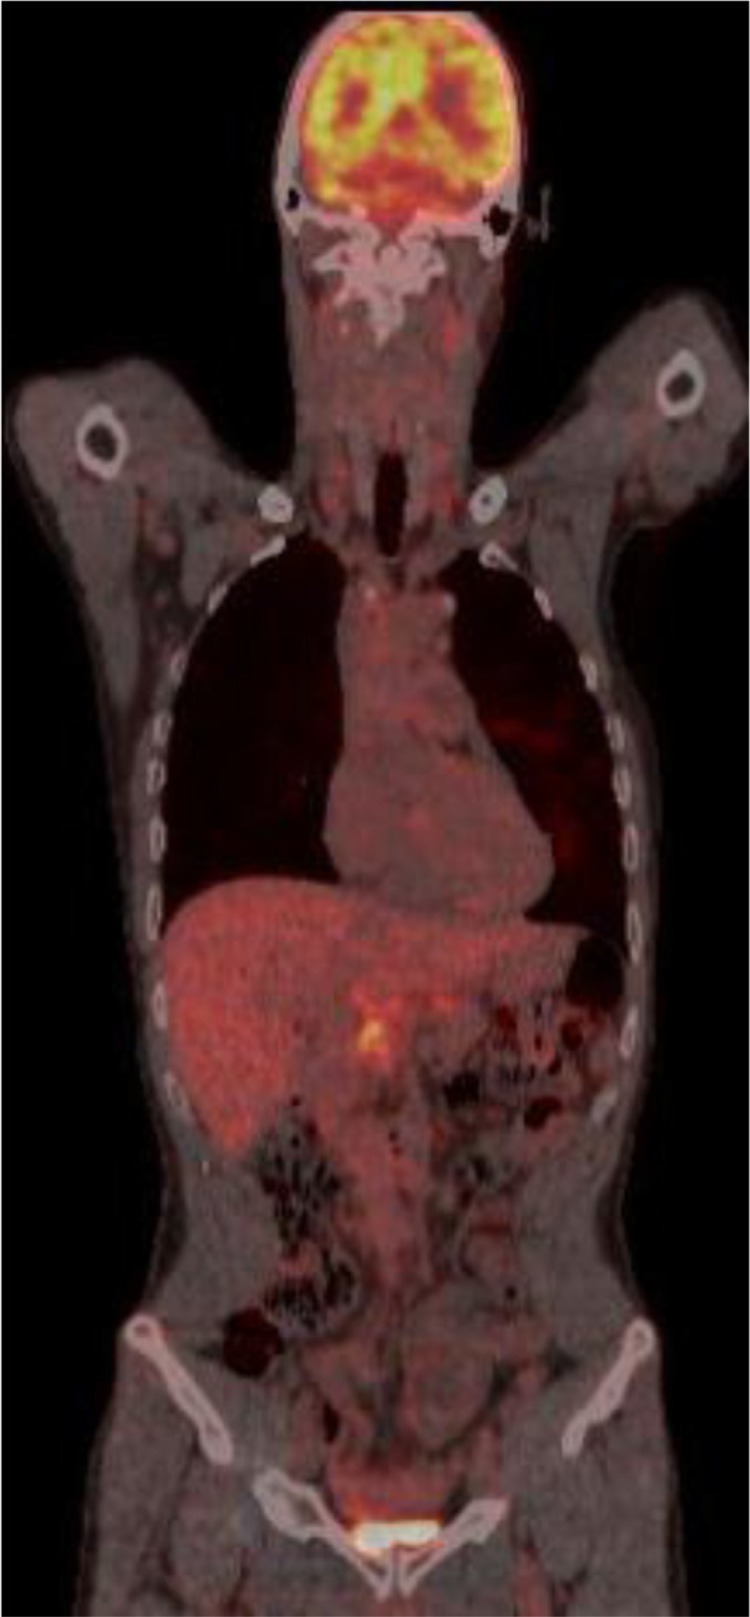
18-FDG PET scanning presenting multiple pathological lesions in lungs, pancreas, lymph nodes, and bones.
